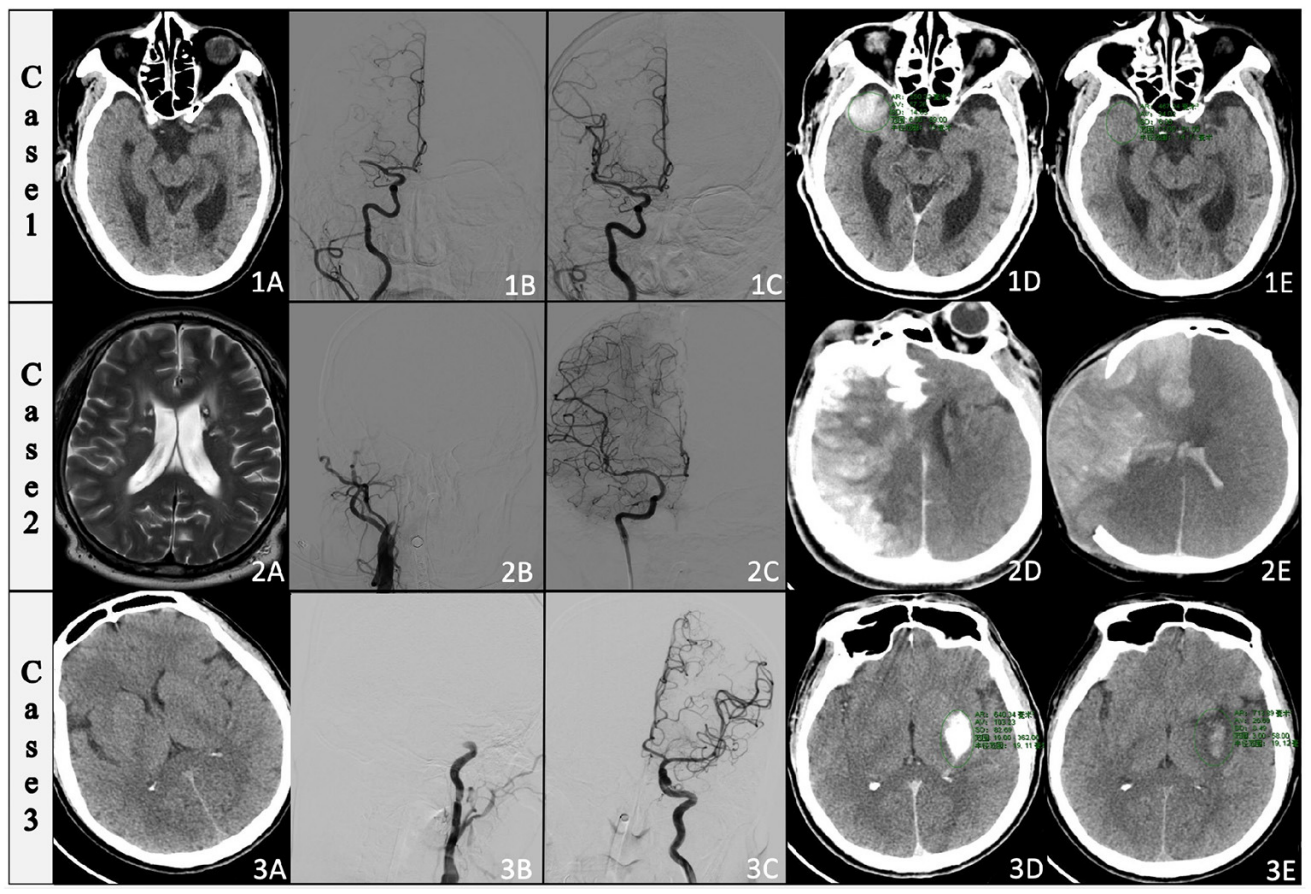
Examples of HALs in patients with acute large vessel occlusion in anterior circulation. CASE-1 demonstrates extravasation of iodine: (1A) Head CT before ET, (1B) DSA showed right M1-segment occlusion that was successfully recanalized (1C). On immediate CT (1D), there were HALs in the right temporal lobe and the highest CT value was 89 HU. The HALs on follow-up CT performed at 24 h after thrombectomy (1E) had vanished. CASE-2 shows IH after thrombectomy: (2A) Head CT before ET and DSA demonstrated right proximal internal carotid artery occlusion (2B) that was recanalized after endovascular treatment (2C). The immediate CT showed a large amount of HALs in the right hemisphere (2D). We measured the highest CT value in the HALs territory to be 413 HU. The follow-up CT showed hemorrhage in the right cerebral hemisphere on the 3 days (2E). CASE-3 shows minor IH after thrombectomy: (3A) Head CT before ET and DSA showed distal occlusion of the internal carotid artery (3B) and was successfully recanalized (3C). The immediate CT (3D) showed HALs in the left ganglia with the highest CT value of 362 HU. The hemorrhage showed in the follow-up CT (3E) was examined at 24 h.
